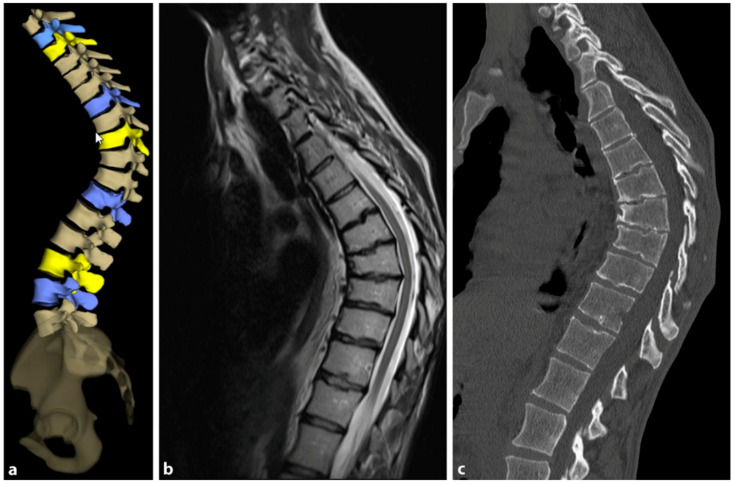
(a) Stereoradiographic imaging, (b) magnetic resonance imaging (MRI) and (c) computer tomography (CT) images of thoracic Scheuermann’s disease (reprinted with permission [23]).
